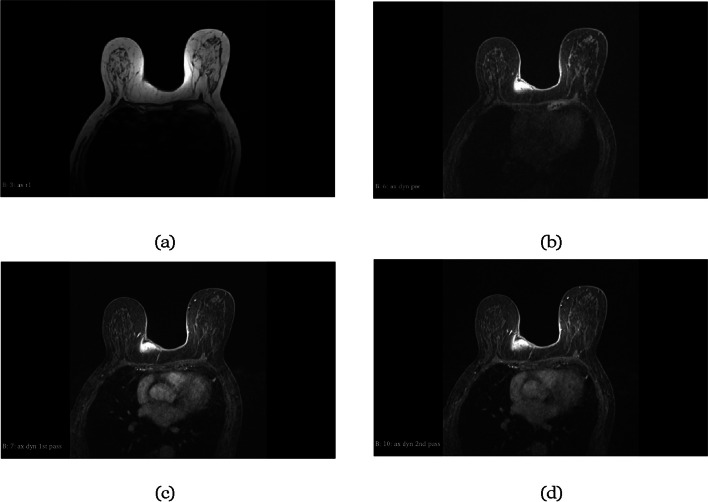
Preoperative DCE-MRI breast tumors from Saha et al. [23]. Example cases of DCE tumor images. a Nonfat-saturated T1-weighted sequence. b Fat-saturated gradient echo T1-weighted pre-contrast sequence. c 1st post-contrast sequence. d 2nd post-contrast sequence
